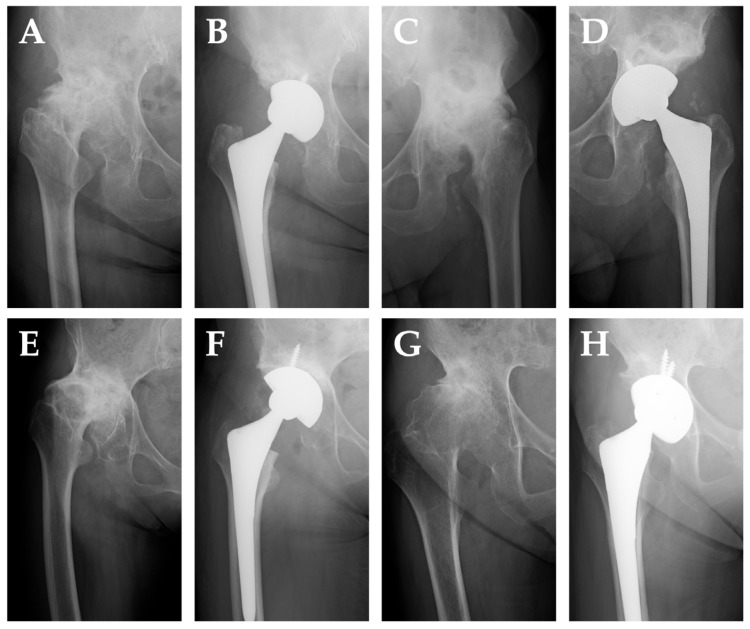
Representative anteroposterior radiographs of total hip arthroplasty (THA) cases. (A,B) A 62-year-old woman who underwent THA 17 years after periacetabular osteotomy (PAO). (C,D) A 66-year-old man who underwent THA 32 years after shelf procedure. (E,F) A 67-year-old woman who underwent THA 25 years after Chiari osteotomy. (G,H) A 68-year-old woman who underwent primary THA without a history of pelvic osteotomy.
